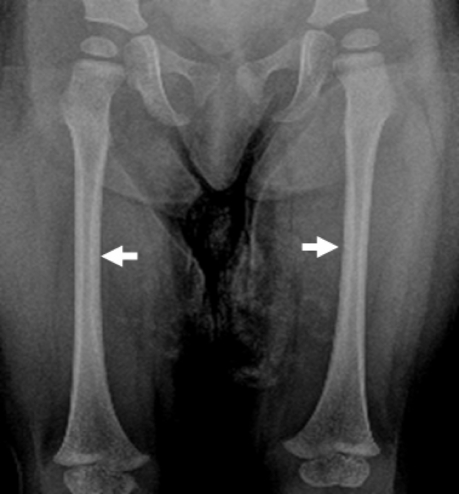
Anteroposterior pelvic-femoral radiograph showed coxa valga associated with mid-diaphyseal endosteal thickening with subsequent medullary narrowing compatible with defective endosteal resorption (arrows).
